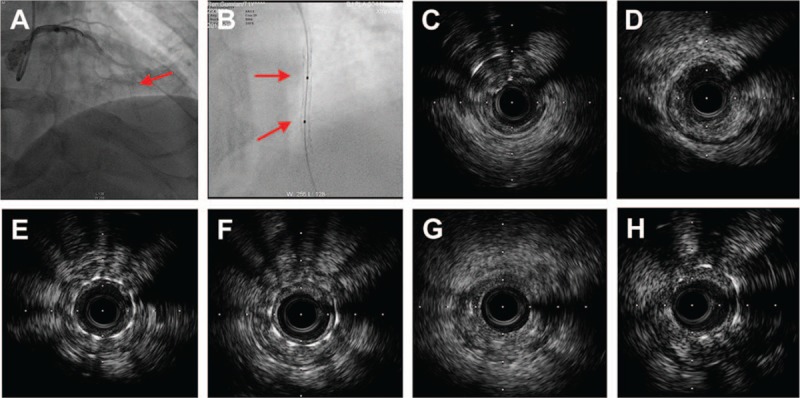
A, The angiogram showed that the old stent in the distal LAD artery was discontinuous (red arrow). B, StentBoost showed that the stent was divided into 3 sections with 2 fractures (red arrow). C–H, The IVUS images showed that the distal segment of the guidewire was in the false lumen (C), and the stent struts disappeared at 2 places (D, G). IVUS = intravascular ultrasound, LAD = left anterior descending.
